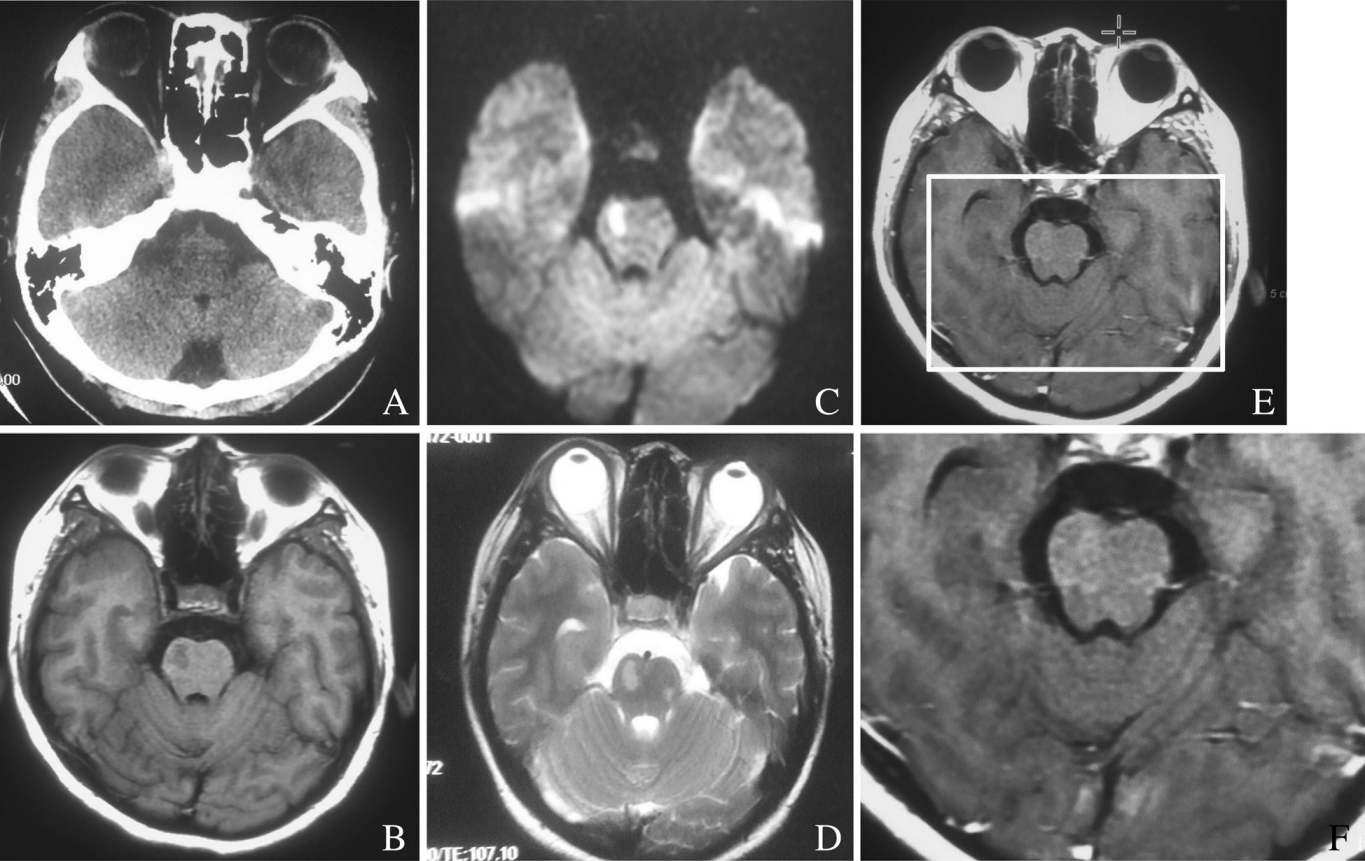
(A) A suspect low-density lesion in the brainstem with no abnormal calcification. (B-F) A brain magnetic resonance imaging scan revealed an egg-like lesion in the brainstem, with a slight hyperintensity in T1, hyperintensity in T2 and DWI, and conglomerates of multiple ring-like enhancements in contrast magnetic resonance imaging.
